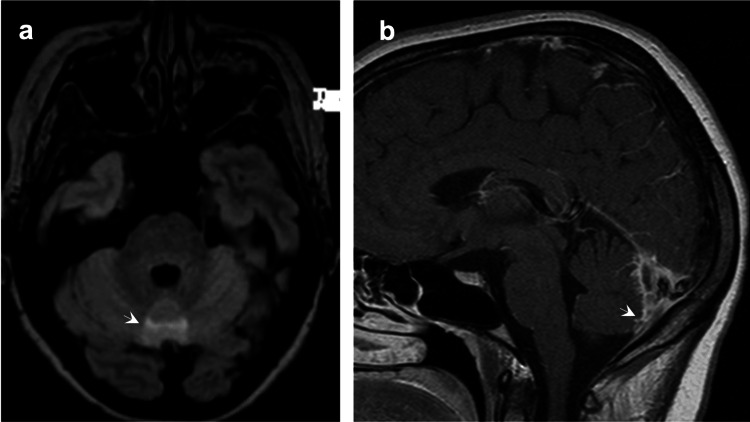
(a) T2WI-FLAIR shows a subtle area of hyperintensity in the posterior region of the cerebellar vermis (arrow). There is no evidence of restricted diffusion, suggesting probable vasogenic edema. (b) Contrast-enhanced T1WI reveals pachymeningeal diffuse thickening and enhancement in the falcotentorial posterior region (arrow). T2WI: T2-weighted imaging; FLAIR: fluid-attenuated inversion recovery; T1WI: T1-weighted imaging.
